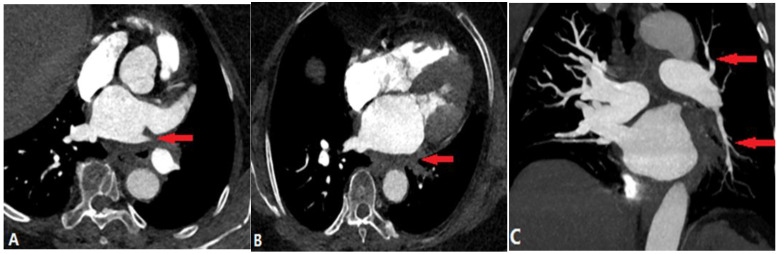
Radiofrequency ablation of atrial fibrillation. (A): Axial CTA shows beak-like narrowing of the left superior pulmonary vein (arrow). (B): Axial CTA shows the occluded inferior pulmonary vein (arrow). (C): Coronal MIP shows the narrowing of left pulmonary arteries due to decreased blood flow (arrows). MIP—maximum-intensity projection.
